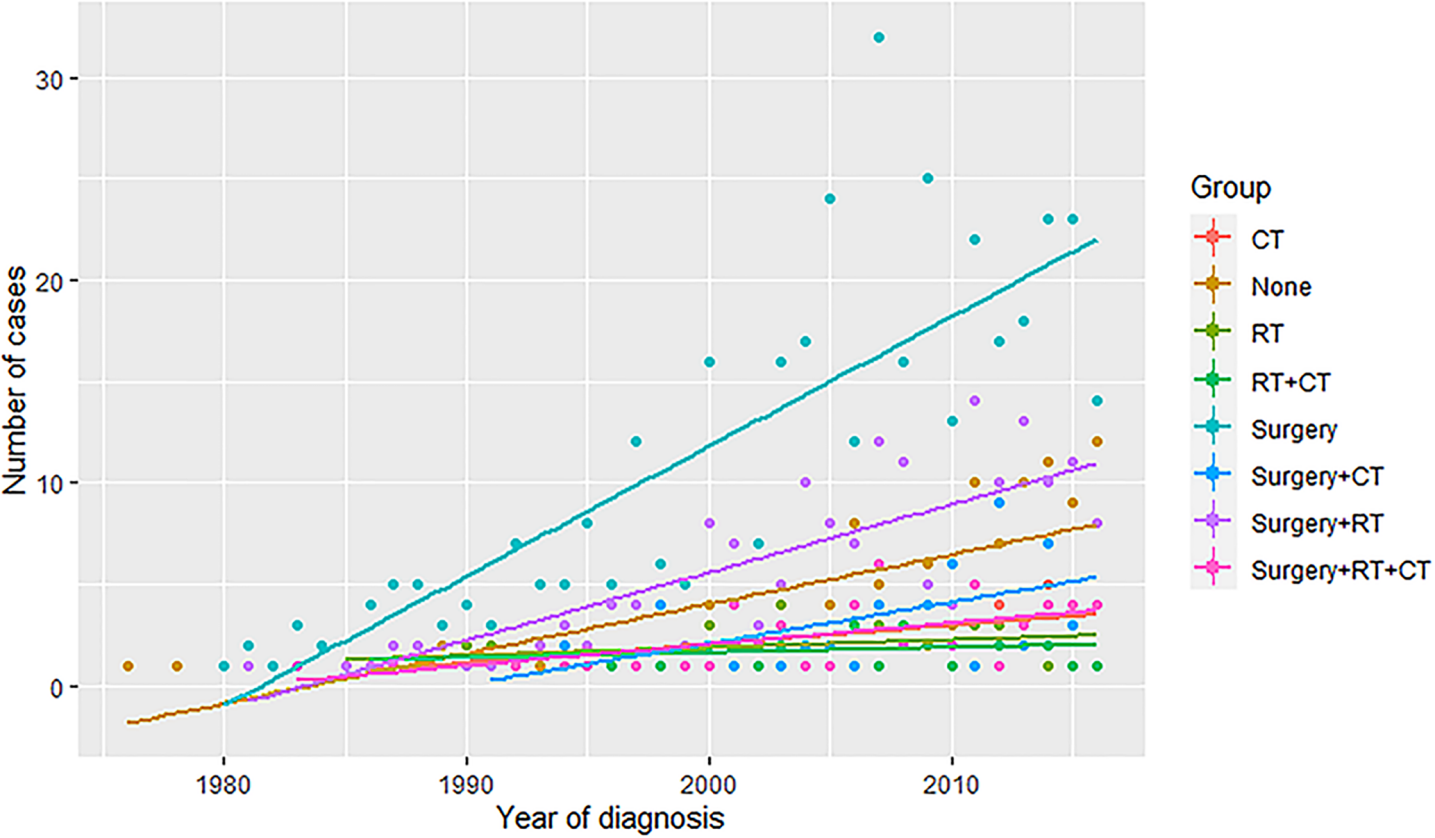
The trends of therapies based on the number of patients who received the same therapy each year. RT, radiotherapy; CT, chemotherapy.
